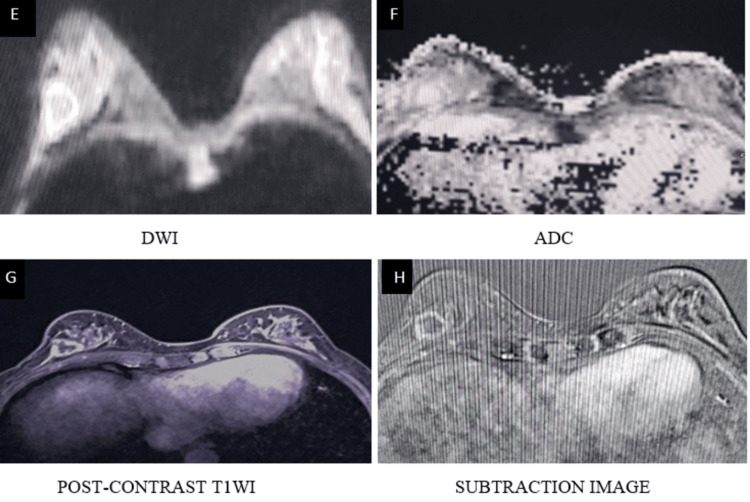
The lesion shows peripheral rim diffusion restriction (E, F) and rim enhancement (G, H) on post-contrast imaging DWI: diffusion-weighted imaging; ADC: apparent diffusion; T1WI: T1-weighted image
